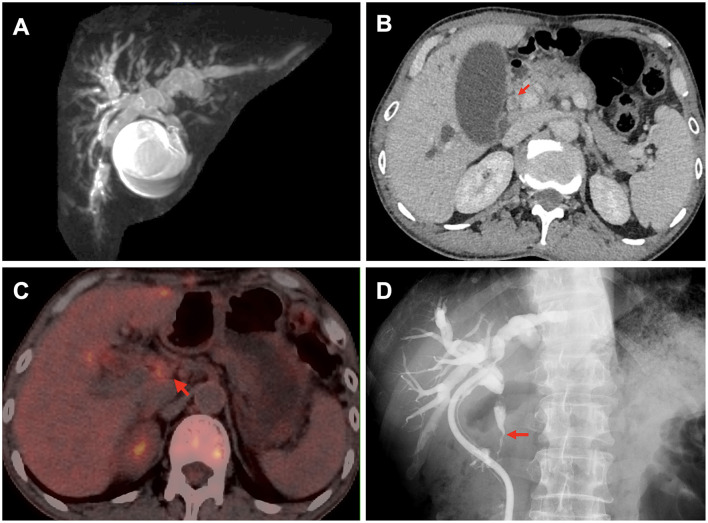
Multimodal imaging findings of the indeterminate biliary stricture. (A) Baseline MRCP showing marked intrahepatic bile duct dilatation; the imaging report described focal wall thickening and focal luminal narrowing in the mid common bile duct. (B) Contrast-enhanced abdominal CT showing a focal stenotic or mass-like lesion involving the mid-to-distal common bile duct region with upstream biliary dilatation (red arrow). (C) FDG PET/CT showing increased uptake in the hilar/biliary region; the early SUVmax was 3.9 and the delayed SUVmax was 5.0 (red arrow). (D) T-tube cholangiography after corticosteroid exposure showing persistent segmental common bile duct stenosis with marked hilar and intrahepatic bile duct dilatation (red arrow).
